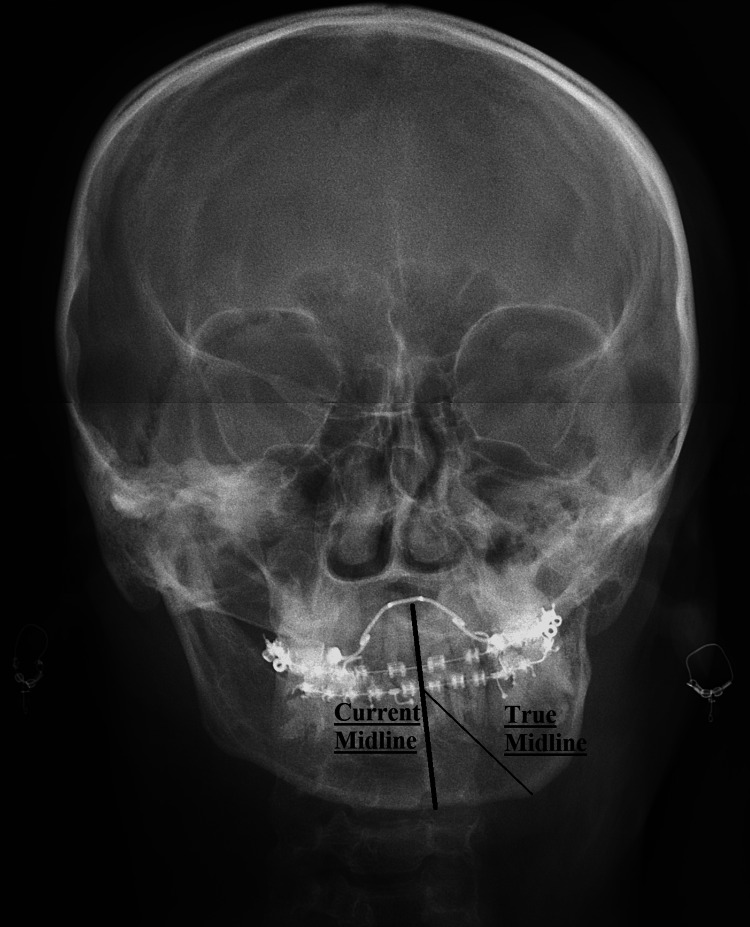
Pre-operative skull X-ray (posteroanterior view) showing shift of the true midline (point B: gnathion on cephalometry) toward the affected side on the mandible
